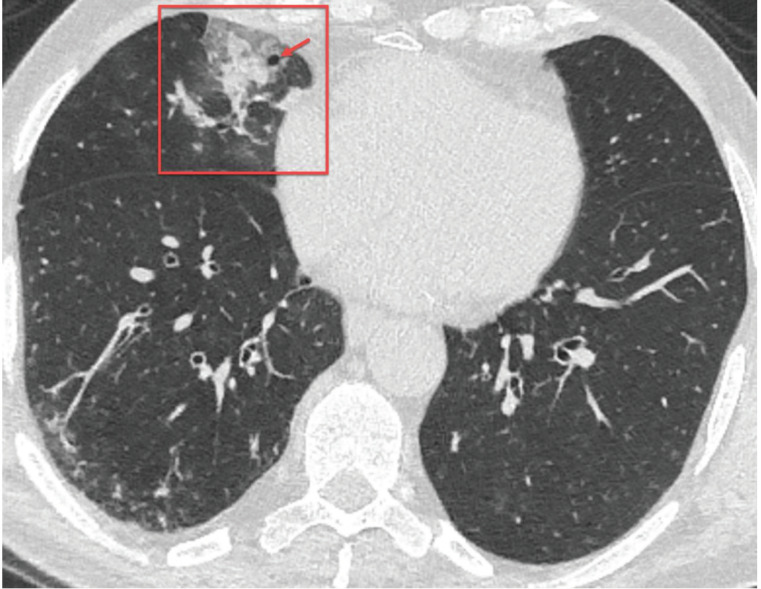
A 75-year-old symptomatic man with COVID-19. Axial chest CT image shows mixed opacity (ground-glass opacity with consolidation) area in the right middle lobe (red frame), and that includes cystic air space (air bubble, red arrow).
